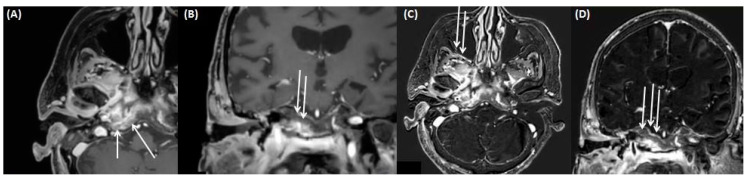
A 84-year-old female patient after mastoidectomy and signs of inflammation of the petrous apex and the skull base with involvement of the paths of the trigeminal and facial nerves, as shown on axial (A) and coronal (B) contrast-enhanced T1-weighted images, provided together with axial (C) and coronal (D) subtraction images (as derived from non-contrast-enhanced and contrast-enhanced T1-weighted images).
